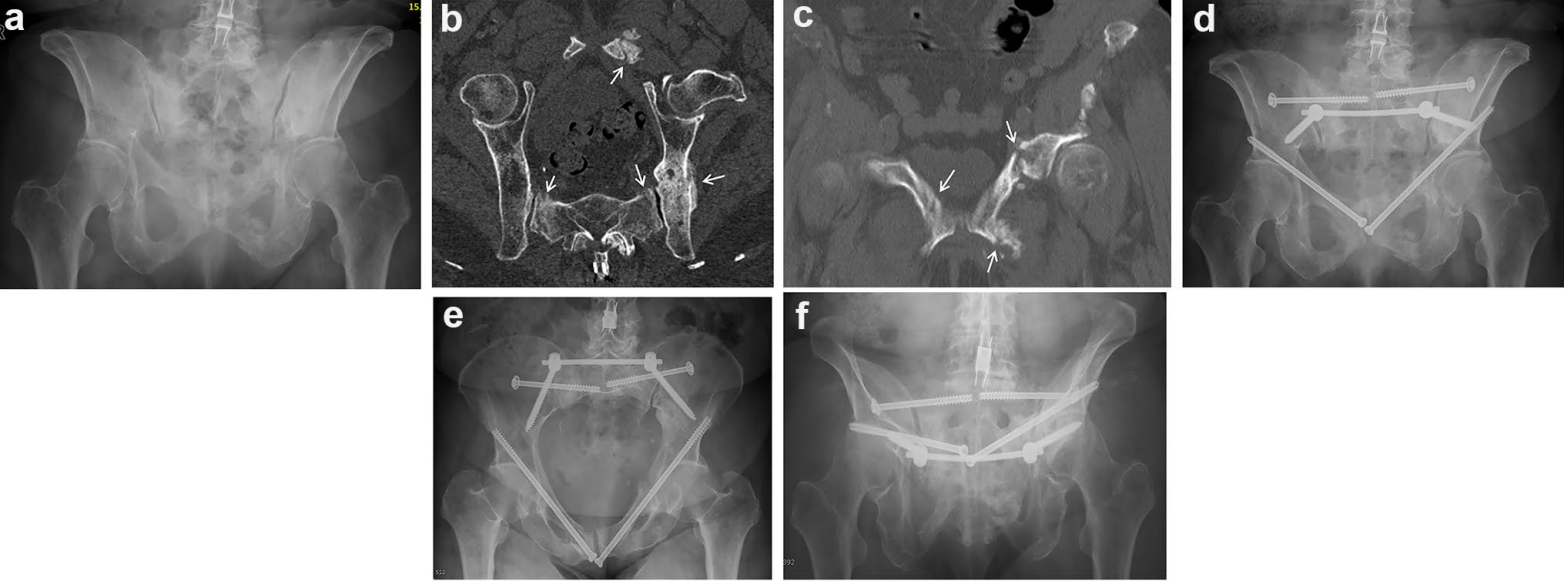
a–f A seventy-three-year-old female suffered of chronic pain in the pelvic region after a fall. There has been a conservative treatment for several months with pain therapy and mobilization. The a.p. pelvic overview after 6 months shows bilateral anterior pubic rami fractures with callus formation but without healing (a). The CT-reconstruction in the plane of the pelvic brim showed bilateral sacral ala fractures, a healed left posterior ilium fracture and a left-sided anterior instability (arrows) (b). Coronal CT-cut through the anterior pelvic ring showed bilateral anterior pubic rami fractures with callus formation but without healing (arrows) (c). These fractures corresponded with a FFP type IVc. Postoperative a.p. pelvic overview (d). The posterior instabilities were fixed with a transiliac internal fixator and bilateral iliosacral screws in S1. There was not enough place for safe placement of a transsacral bar in S1. The anterior instabilities were transfixed with two retrograde transpubic screws. Postoperative pelvic inlet view (e). Postoperative pelvic outlet view (f)
